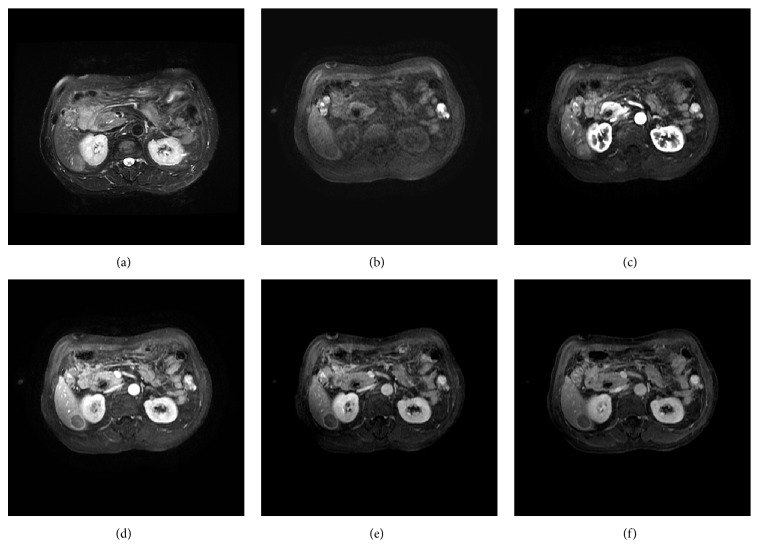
Axial MR images and pathologic image of a 70-year-old man with HCC. (a) A fat-suppressed T2-weighted fast spin-echo image shows an oval-shaped, slightly hyperintense neoplasm in the dorsal part of segment VI, with a maximum diameter of 2.6 cm. Axial precontrast (b), late artery phase (c), portal vein phase (d), equilibrium phase (e), and delay phase (f) T1-weighted 3D GRE images demonstrate a hypointense appearance of the lesion on precontrast T1-weighted images (b), high enhancement in the late arterial phase, (c) and washout in the portal vein phase (d) with an enhancing capsule that can clearly be observed in the equilibrium phase (e) and delay phase (f); all of these MRI features are consistent with typical HCC. The tumor was successfully surgically resected and was pathologically confirmed as a WD HCC.
